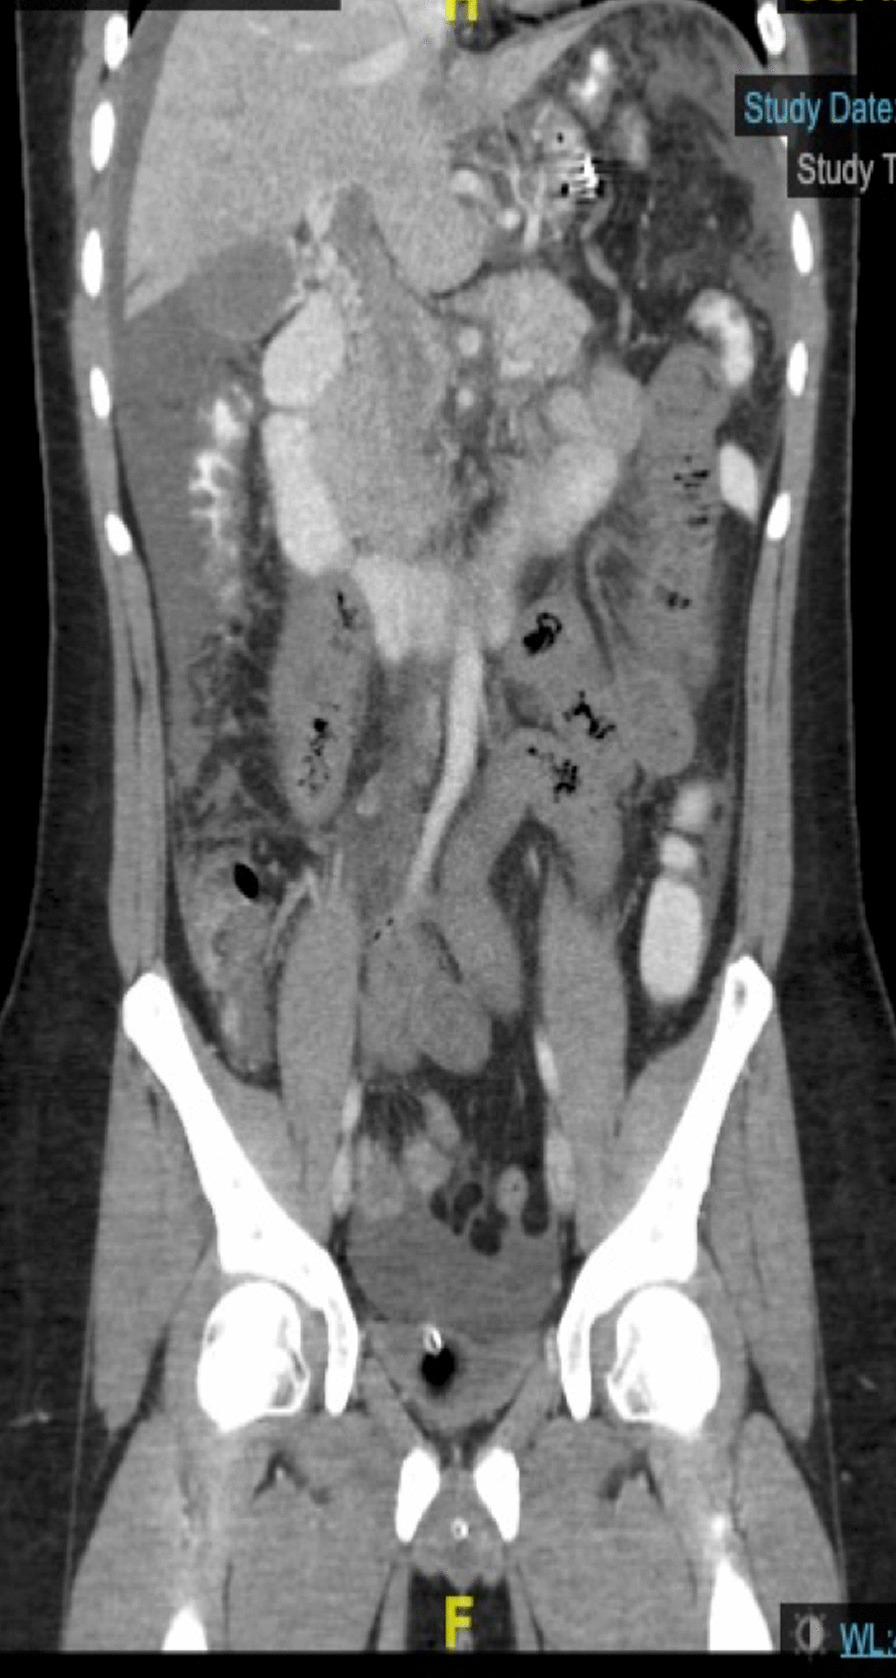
CT abdomen and pelvis demonstrating PVMT and signs of small bowel ischemia
